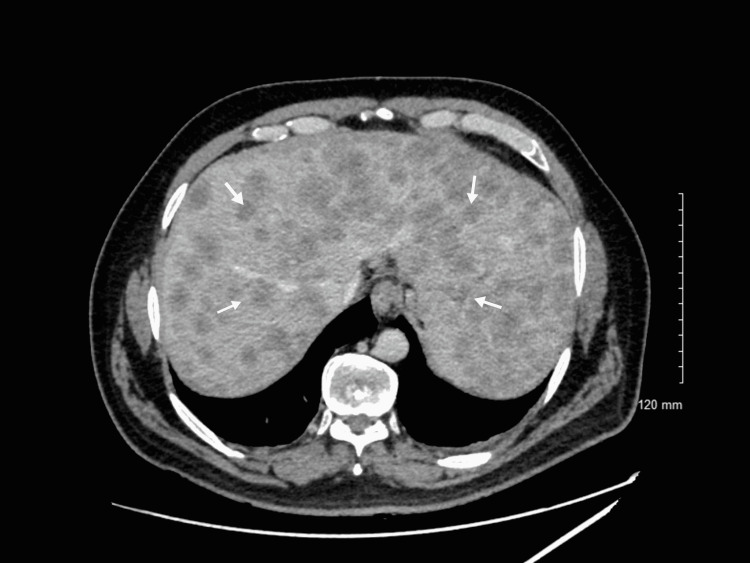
CT scan of the abdomen and pelvis revealing enlarged liver with innumerable low-density lesions occupying half of the liver parenchyma (white arrows).
